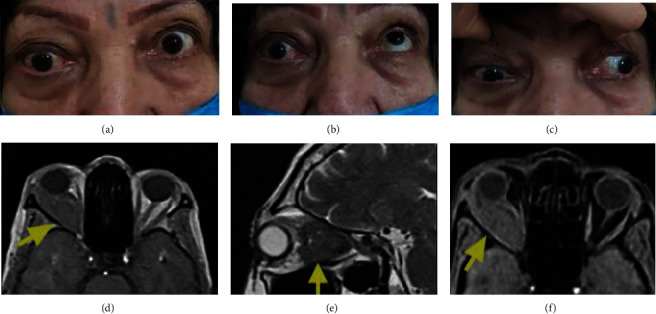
A 45-year-old female presented with proptosis, periorbital fullness, and movement limitation in the right eye (a–c). Orbital MRI demonstrates a large orbital mass in the lateral part of the right orbit that spreads to the orbital apex and pushes the optic nerve medially. The mass is isointense to the brain tissue in T1- (d) and T2-weighted (e) images and enhances homogeneously in T1-weighted fat saturation (f).
